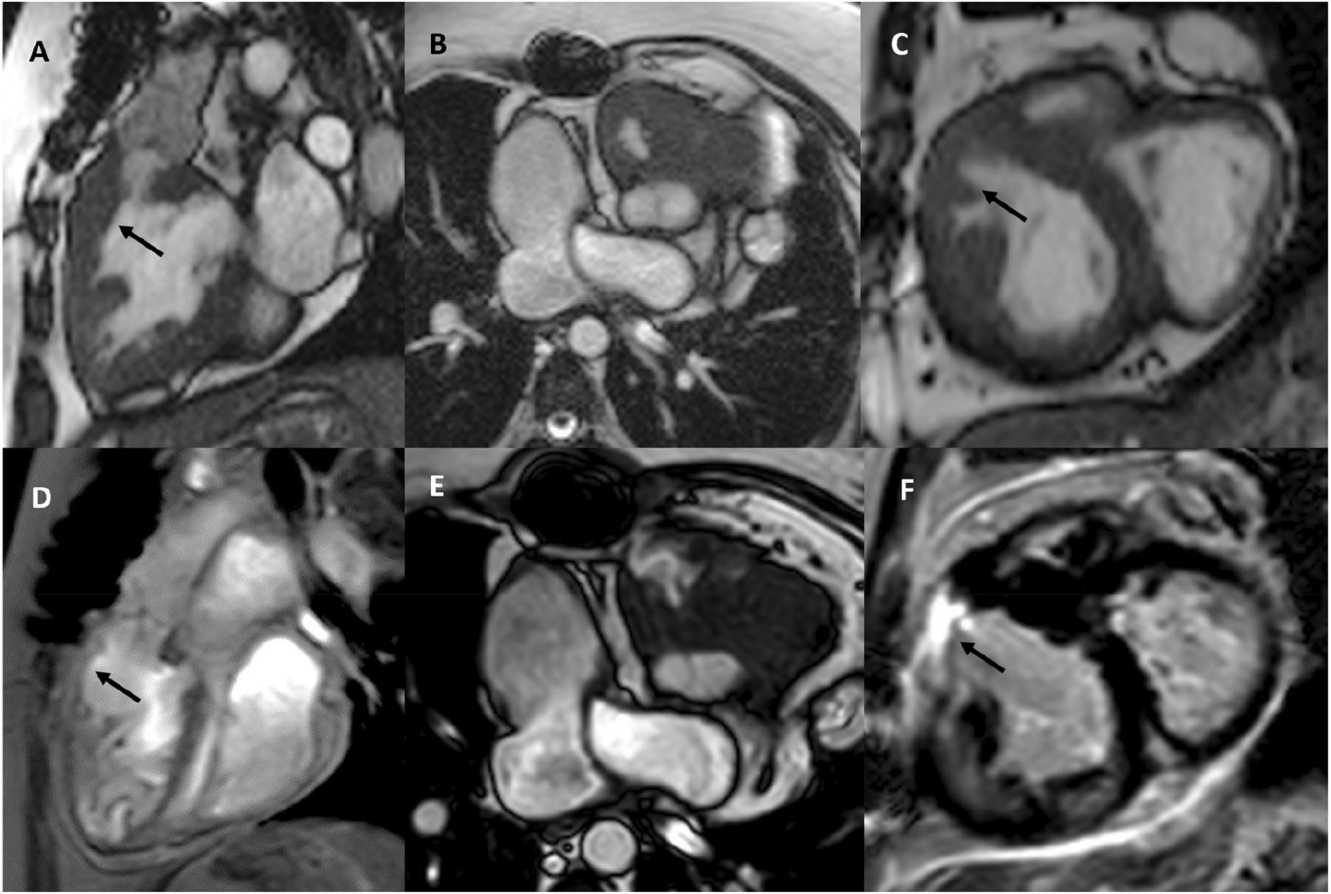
Cardiac magnetic resonance imaging demonstrating relevant right ventricular outflow tract obstruction before the procedure (A–C). Infundibular hypertrophy causing the obstruction is marked with an arrow. After the procedure, the infundibulum appears much thinner compared with the preprocedural images (D, arrow). Late gadolinium enhancement (LGE) depicts scar (arrow) tissue within the right ventricular outflow tract, consistent with the targeted ablation site (E, F).
